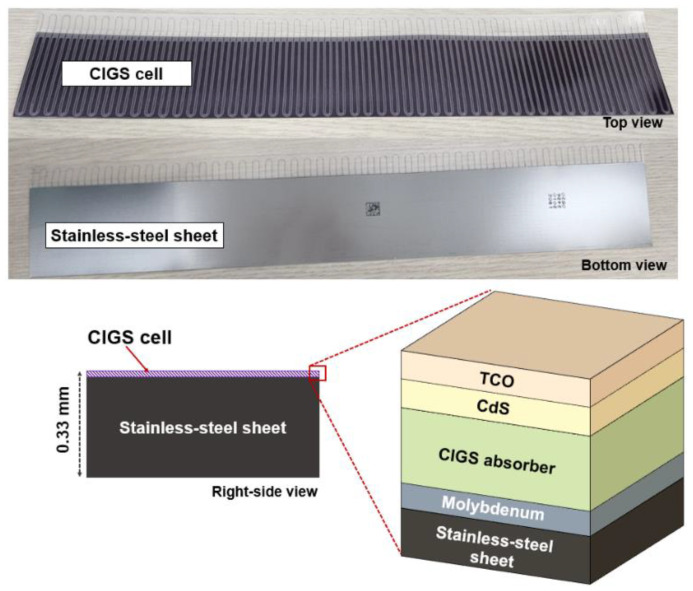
A commercialized copper indium gallium selenide (CIGS) solar cell and its structure. The area comprising the CIGS cell is the active area that converts therapeutic X-ray radiation to electrical signals. TCO and CdS denote transparent conductive oxide and cadmium sulfide, respectively.
